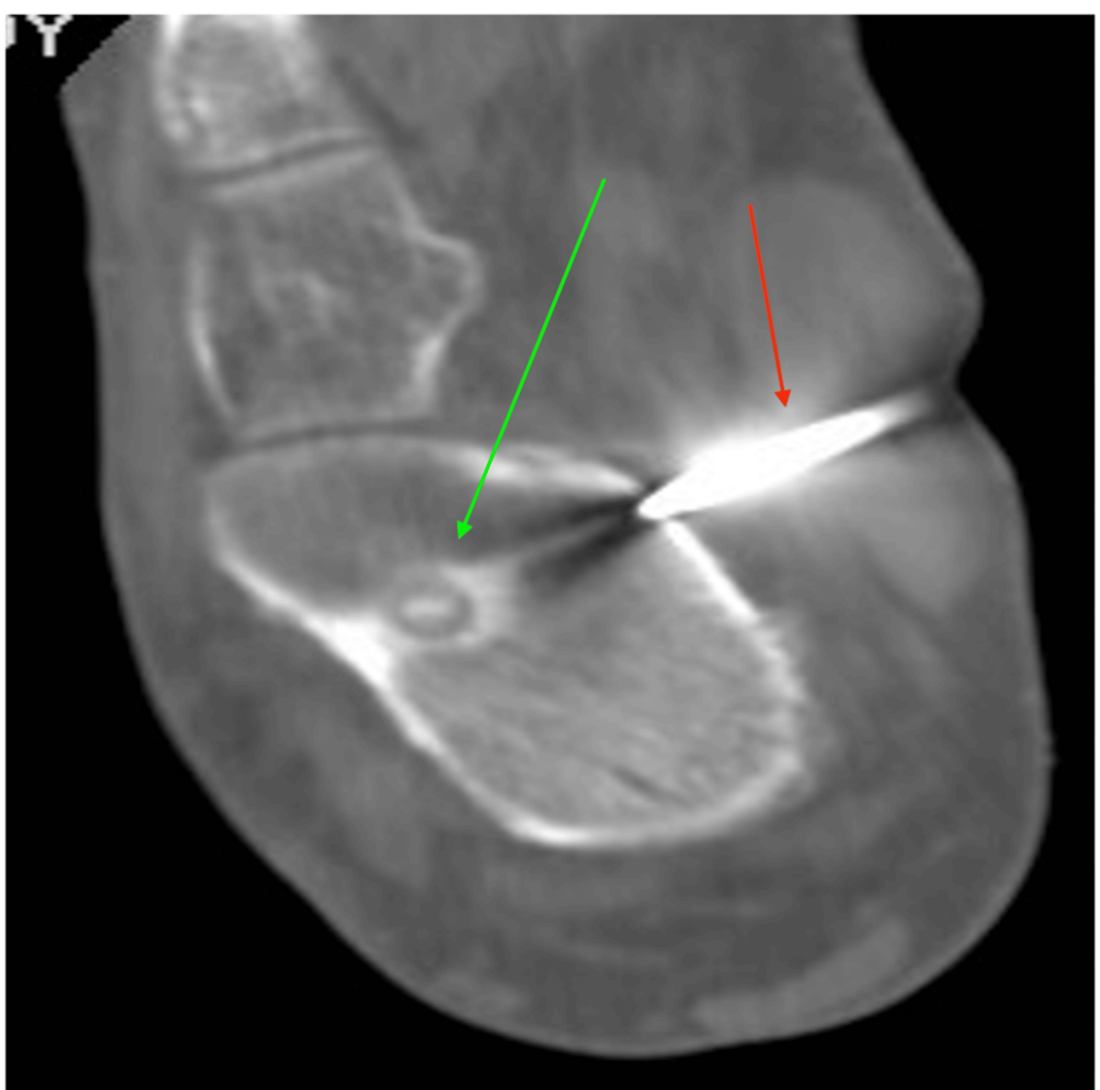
Computed tomography (coronal view)-guided percutaneous radiofrequency ablation (RFA) of the calcaneus The red arrow indicates the needle passing through the skin and soft tissues into the calcaneus. The green arrow points to the well-defined nidus in the anterior and middle regions of the calcaneus.
